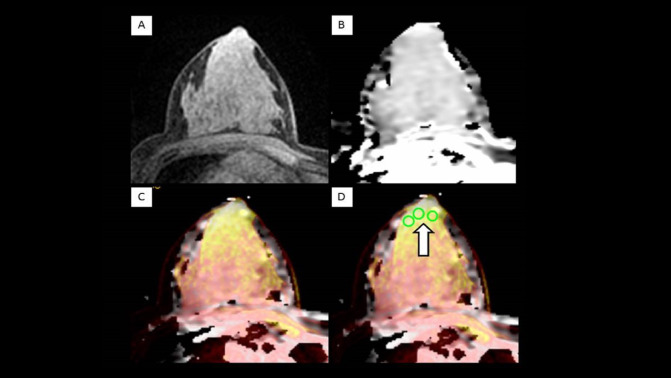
ROI placement for apparent diffusion coefficient (ADC) measurement in normal fibroglandular tissue. (A) T1-weighted fat-suppressed image. (B) Corresponding ADC map of the normal breast. (C) Fusion of T1-weighted fat-suppressed image and ADC map. (D) Single ROI placed in retroareolar fibroglandular tissue, avoiding ducts and vessels. ADC = apparent diffusion coefficient.
